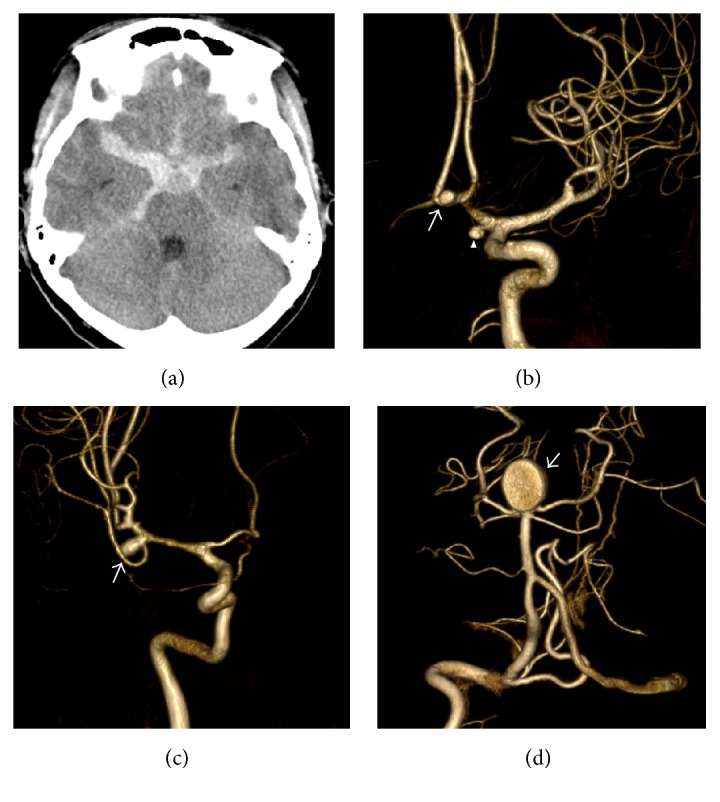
(a) Noncontrast brain CT shows diffuse subarachnoid hemorrhage. Three-dimensional digital subtraction angiography demonstrating anterior communicating artery aneurysm ((b) arrow), A1 segment of left anterior cerebral artery ((b) arrowhead), bifurcation of right middle cerebral artery aneurysm ((c) arrow), and basilar artery bifurcation aneurysm ((d) arrow).
